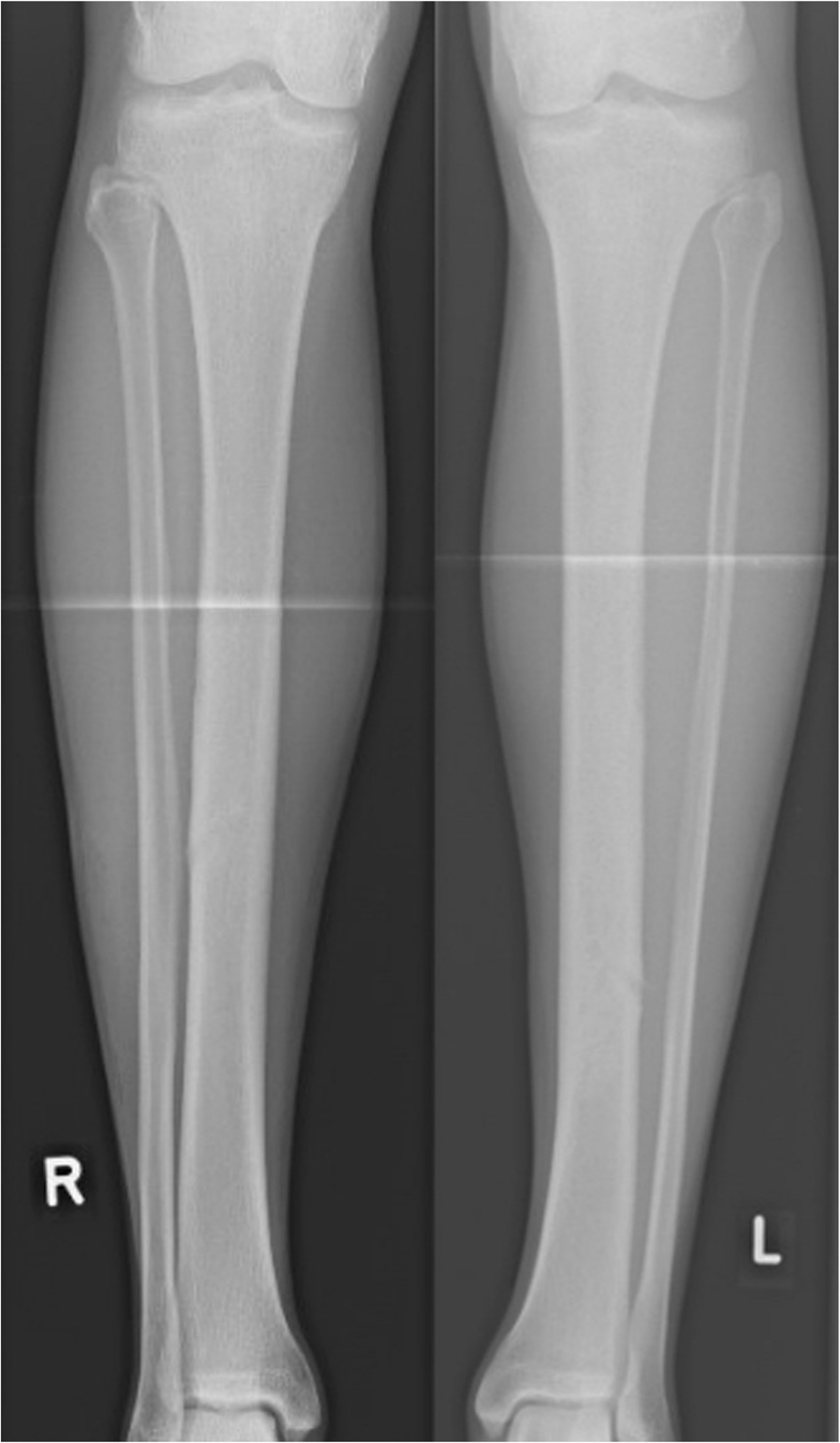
Plain radiography a.p. after percutaneous removal of the cannulated screws (34/31 months after initial surgery)
